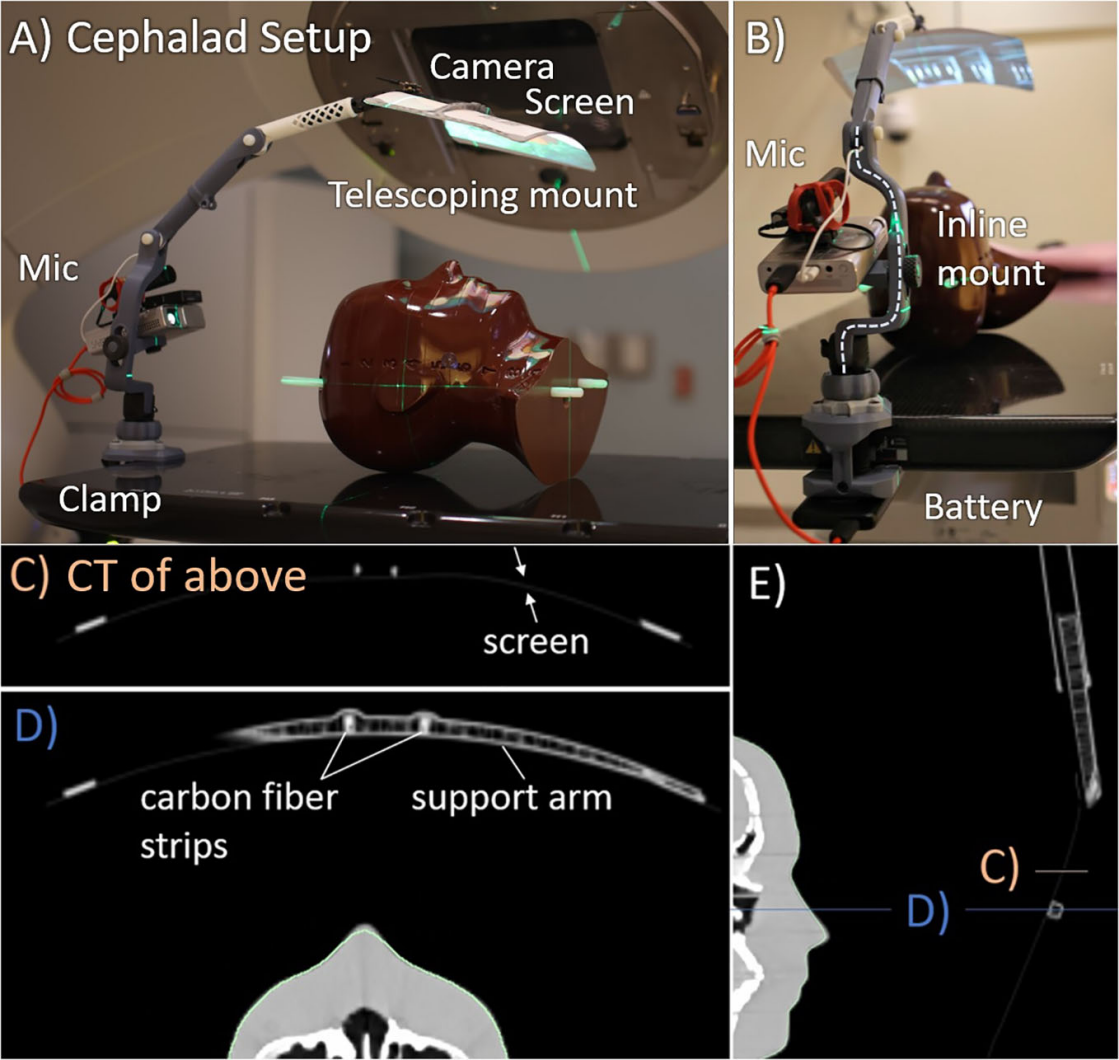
Cephalad AVATAR system setup and CT images. (A) Side view. (B) Superior view, looking down the treatment table. The computed tomography (CT) slices shown in (C–E) have Hounsfield unit (HU) range of −1,000 (black) to 400 HU (white). (C, D) Axial slices through the screen and hollow support arm. The support arm has wall thickness of 0.8 mm and infill fraction less than 10%. Similarly, the telescopic tube shown in (E) also has wall thickness of 0.8 mm and infill fractions of less than 10%.
